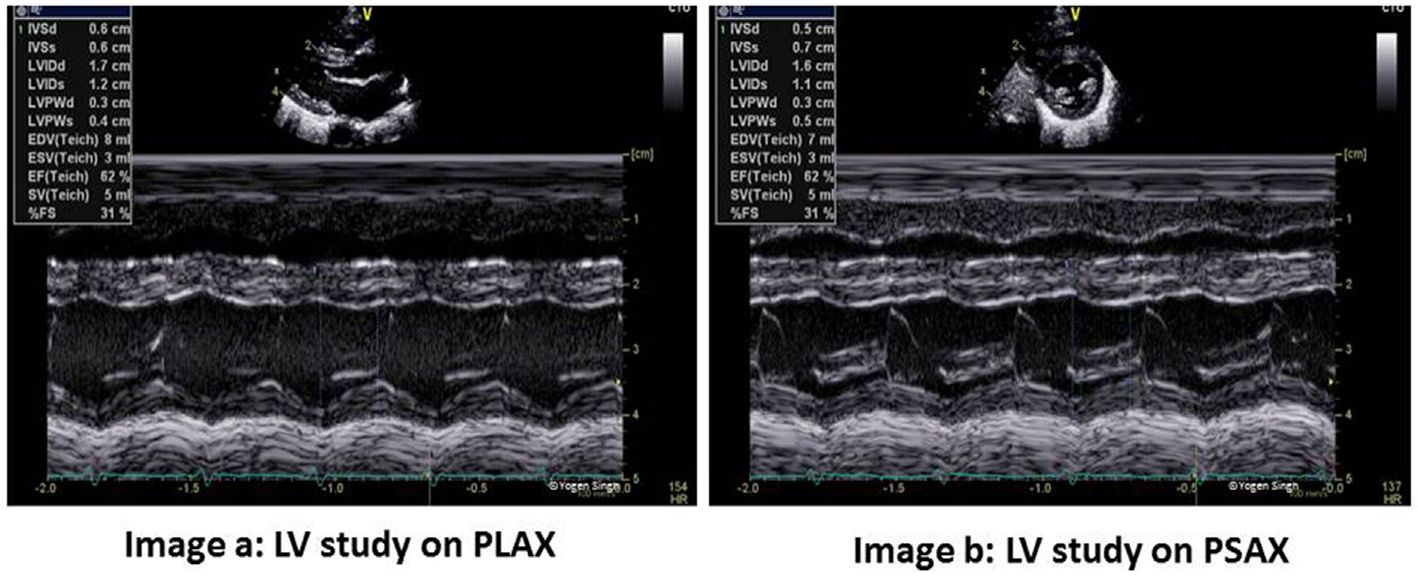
Left ventricle (LV) study in PLAX and PSAX views. FS and EF can be measured reliably on both views - image a shows FS measurement in PLAX and image b shows same measurement in PSAX view. However, EF probably best measured by Simpson’s method. PLAX, parasternal long axis view; PSAX, parasternal short axis view; LV, LV study; FS, fraction shortening; EF, ejection fraction.
